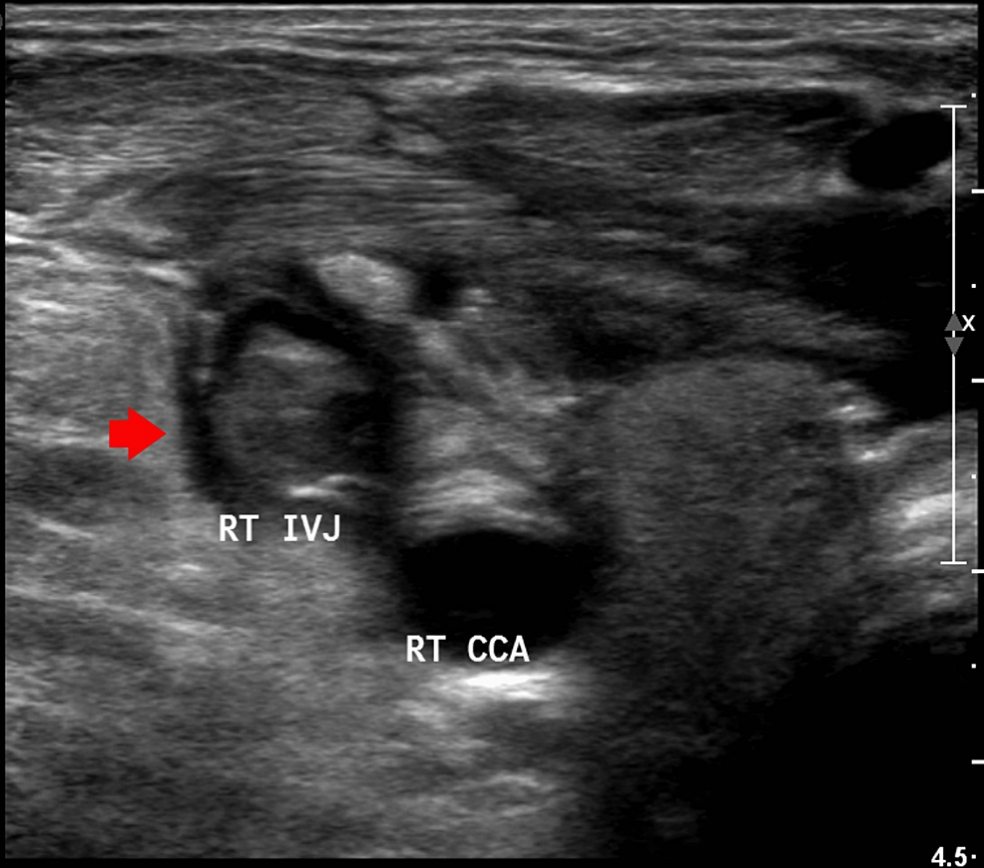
Doppler ultrasonogram of the right internal jugular vein showing echogenicity (red arrow) indicating the presence of thrombus RT IVJ: right internal jugular vein, RT CCA: right common carotid artery
